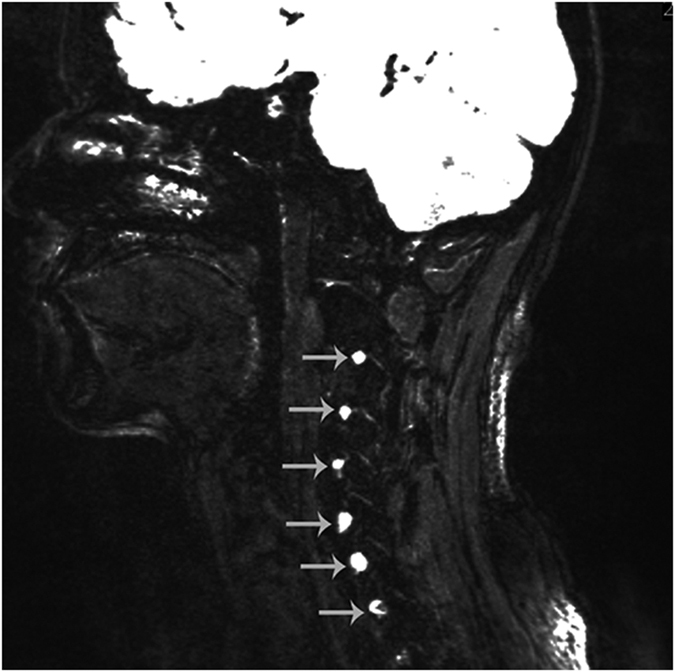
Sagittal view of the MRI, Ganglia C3 through C5 (white arrow) on the right side of the body can be visualized.
